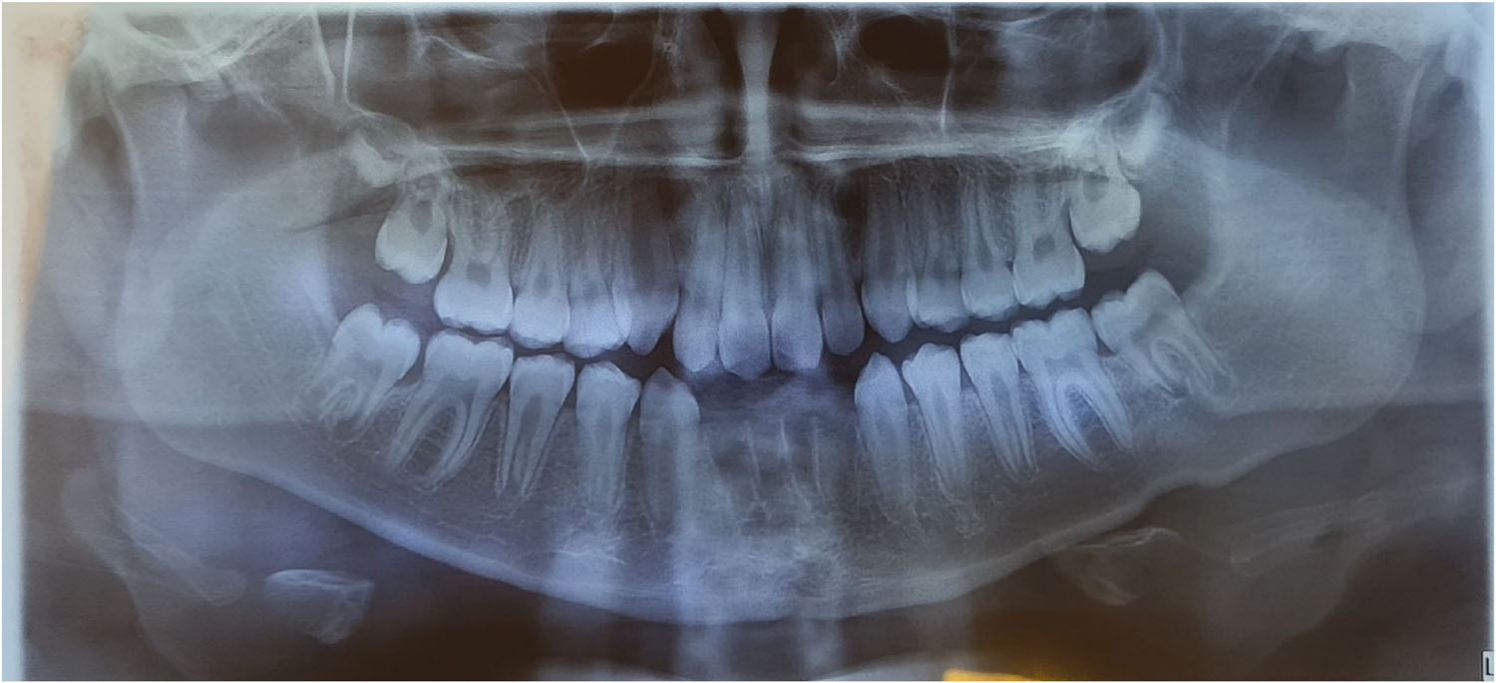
Panoramic radiograph showing recent loss of lower incisors (Case 2).
